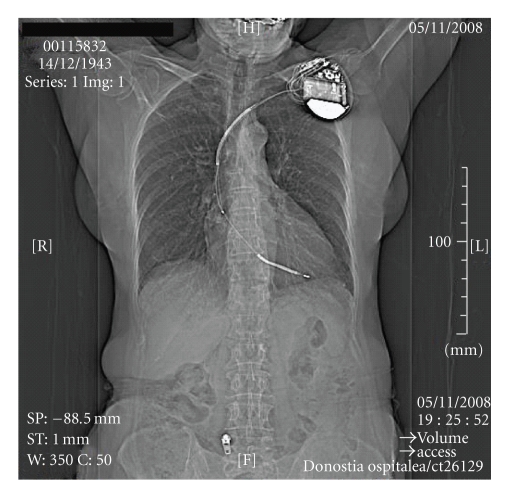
CT topogram. Initial topogram of the scan, showing the implanted device in the left pectoral zone and both electrodes. Arrows: date and time of scan.
